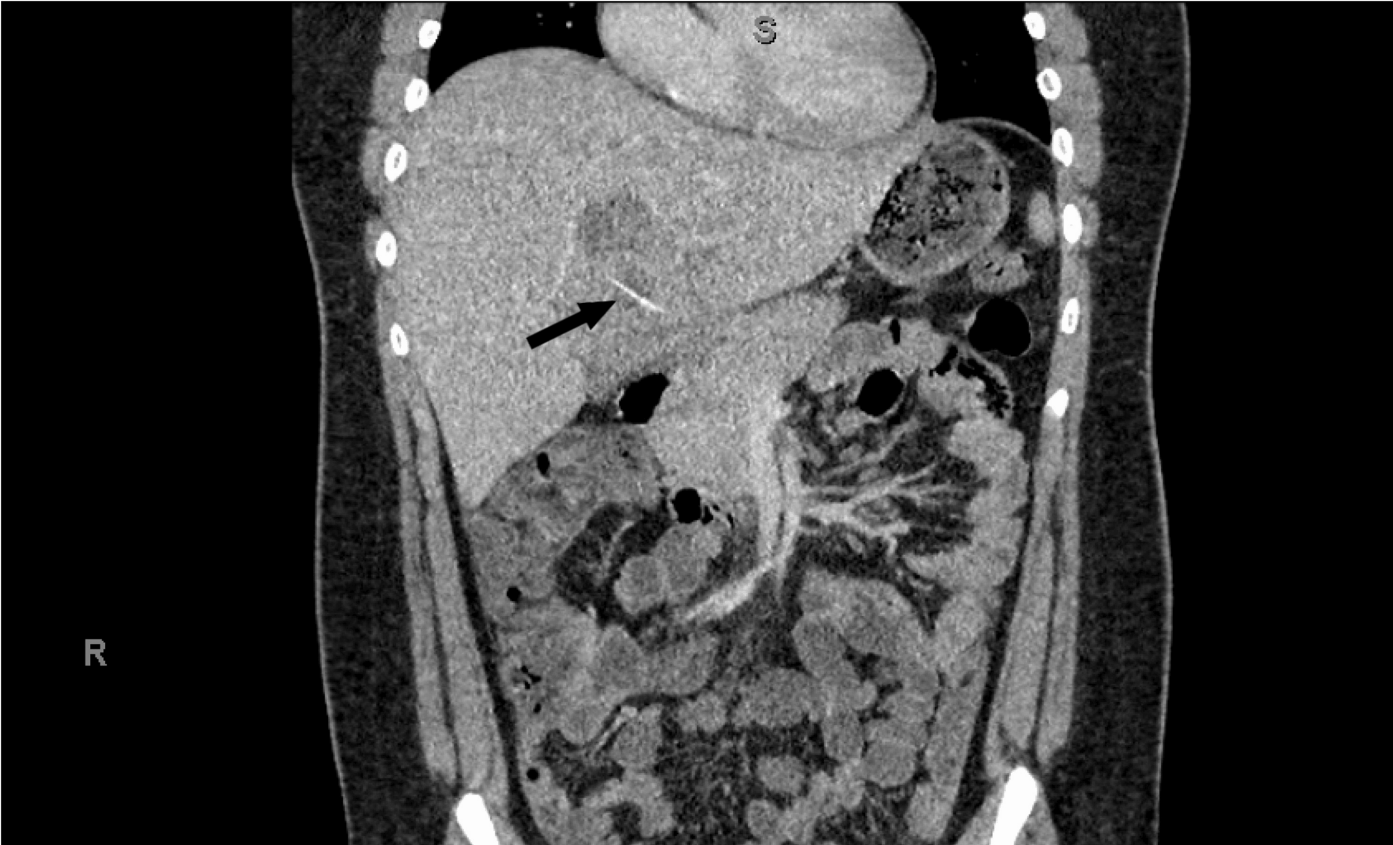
Initial computed tomography (CT) of the abdomen. Initial spiral CT of the abdomen demonstrating an infiltrative heterogeneous hepatic mass thought to be predominately in segment IV. This mass was first characterized to have peripheral calcification, concerning for malignancy. There was no evidence of additional foci of disease within the abdomen. These findings were subsequently amended to include the presence of a thin curvilinear density (black arrow).
